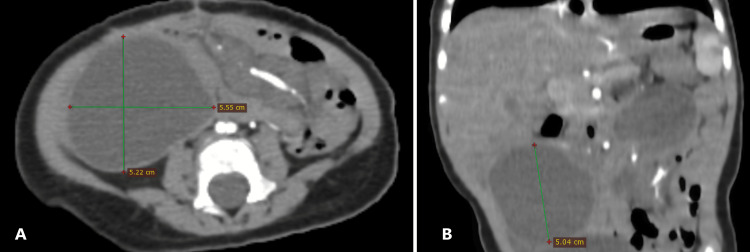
CT scan findings A: axial; B: coronal images of CT showing the enteric duplication cyst adjacent to the volvulus zone.
